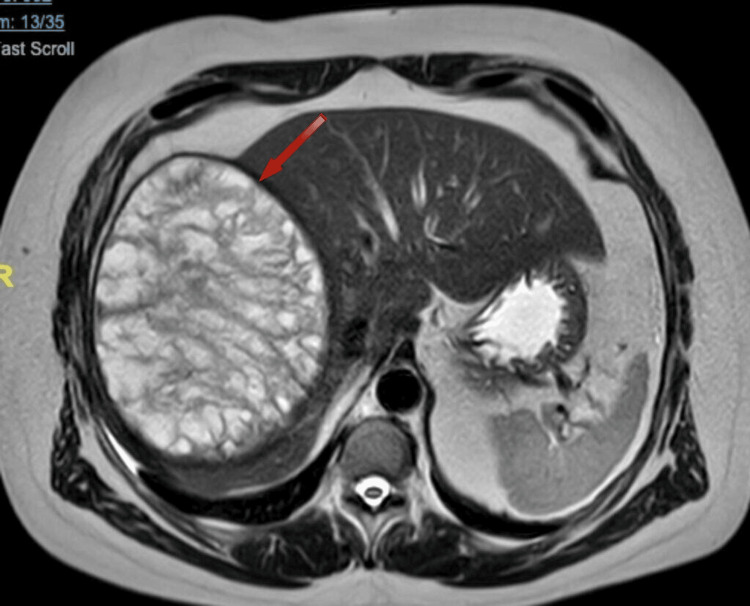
MRI axial plane Numerous irregular septa are clearly visible inside.
